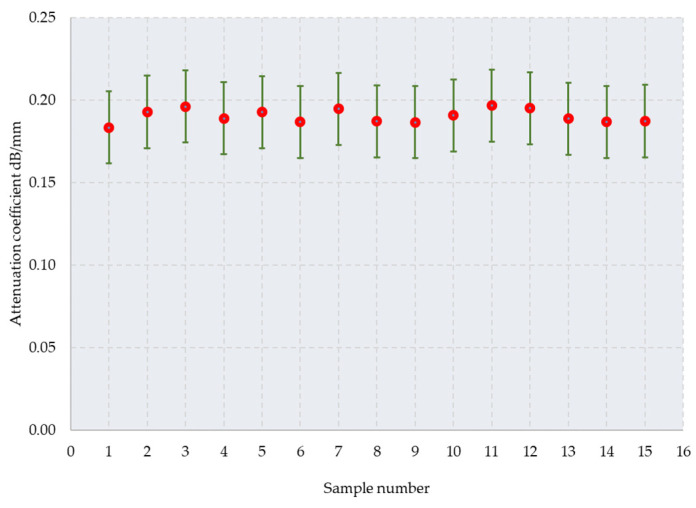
Damping coefficient of ultrasound for all tested samples.
